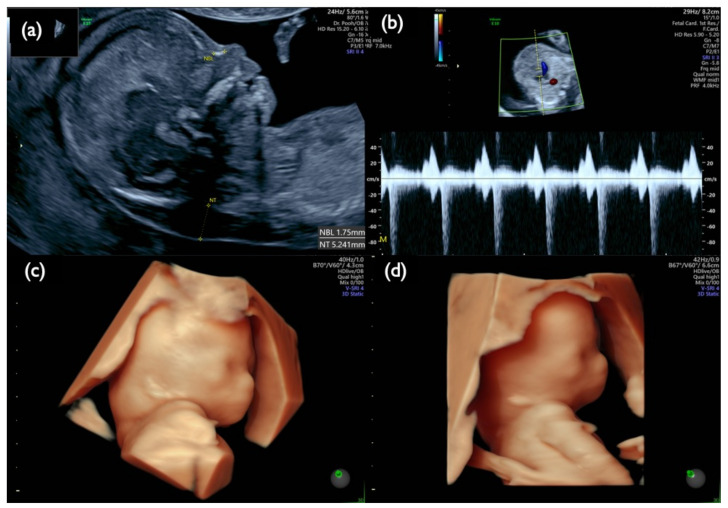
Fetal ultrasound scan of Case Mo4 at 13 weeks. (a) Mid-sagittal section of the fetal head. Nuchal translucency (NT) of 5.2 mm and small nasal bone are demonstrated. (b) Tricuspid regurgitation. (c,d) 3D reconstructed fetal face. A flat profile with a low-set ear and swollen eye bags are clearly demonstrated.
